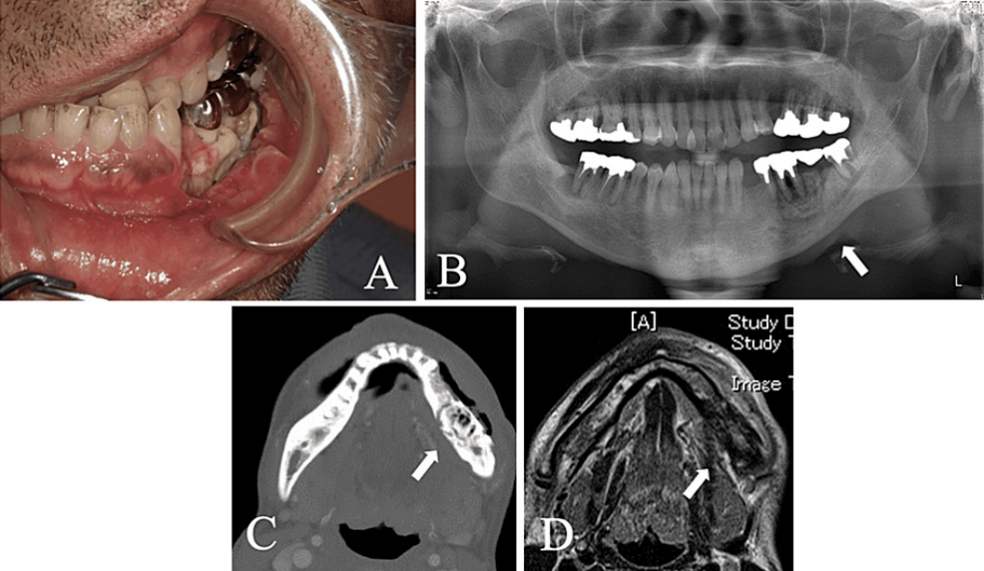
Image findings at initial examination. A: Intraoral examination; A 35mm-sized ulcerative lesion with marginal swelling, bone exposure, and drainage is seen on the lower left 5-8 gingiva. B: Panoramic X-ray findings; rotund-like bone resorption and bone separation in the left lower sixth. C: Contrast-enhanced CT findings (horizontal section); rotting bone-like bone resorption and bone separation in the left mandibular molar region. D: Contrast-enhanced MR findings; T2-weighted image shows abnormal marrow signal in the left mandibular body and uniform thickness of swelling in the buccal gingival region.
